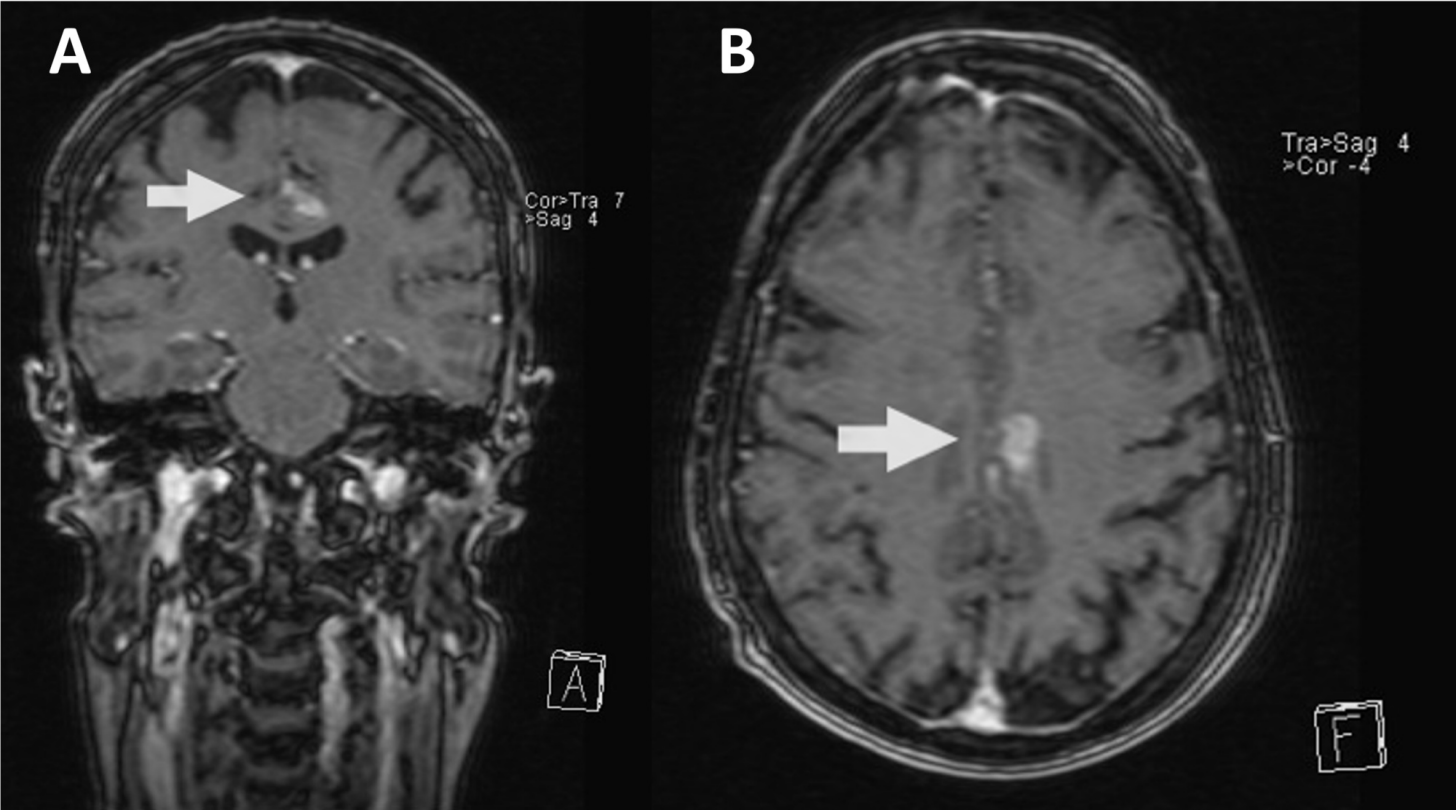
Patient 2 magnetic resonance imaging (MRI) causing division between readers T1-weighted gadolinium enhanced images of candidate lesions causing division between readers (A: Coronal view, B: Axial view).
